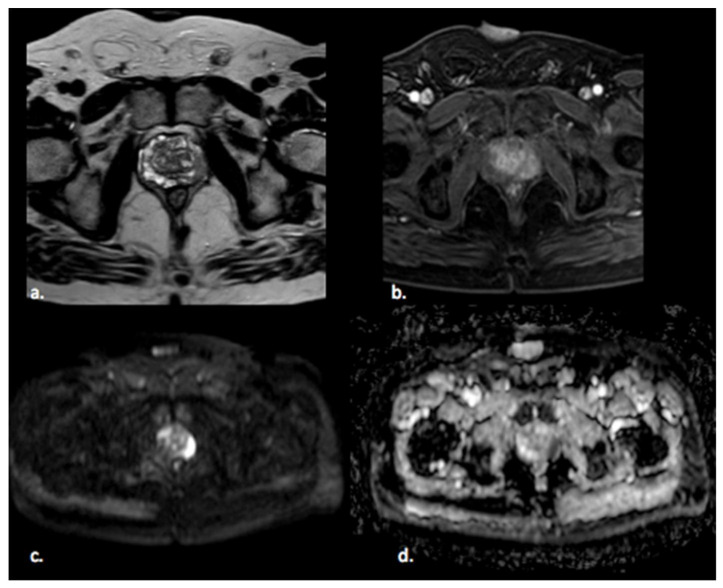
A 65 year old man with a PI-RADS 5 lesion in the left postero-lateral segment of the PZ of midgland, hypointense in T2-weighted images (a), with early enhancement in DCE images (b), markedly hyperintense on DWI, and hypointense on ADC images (c,d).
